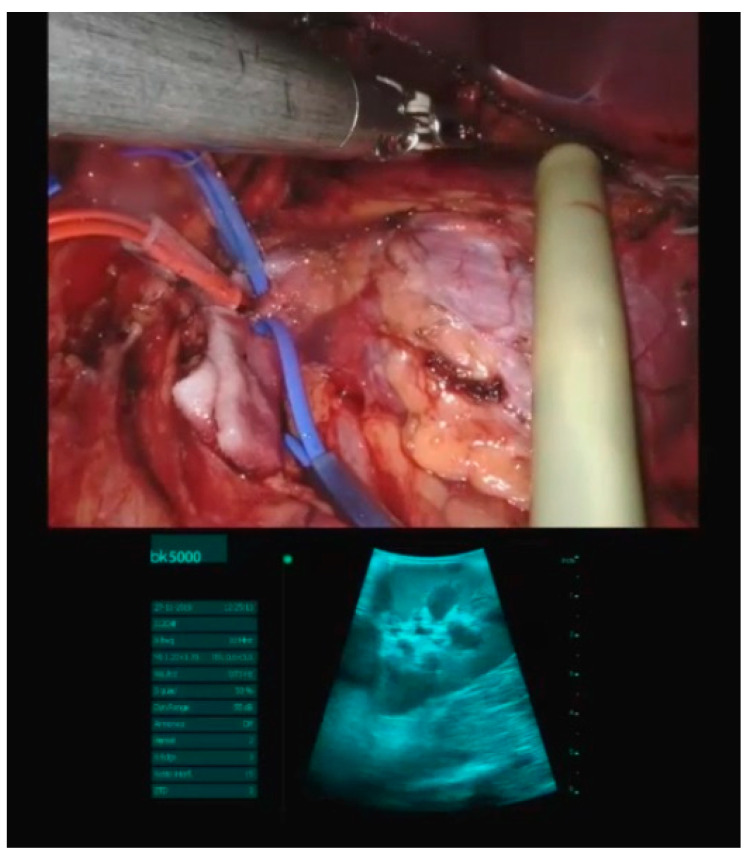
Intraoperative ultrasound during robotic-assisted surgery.
